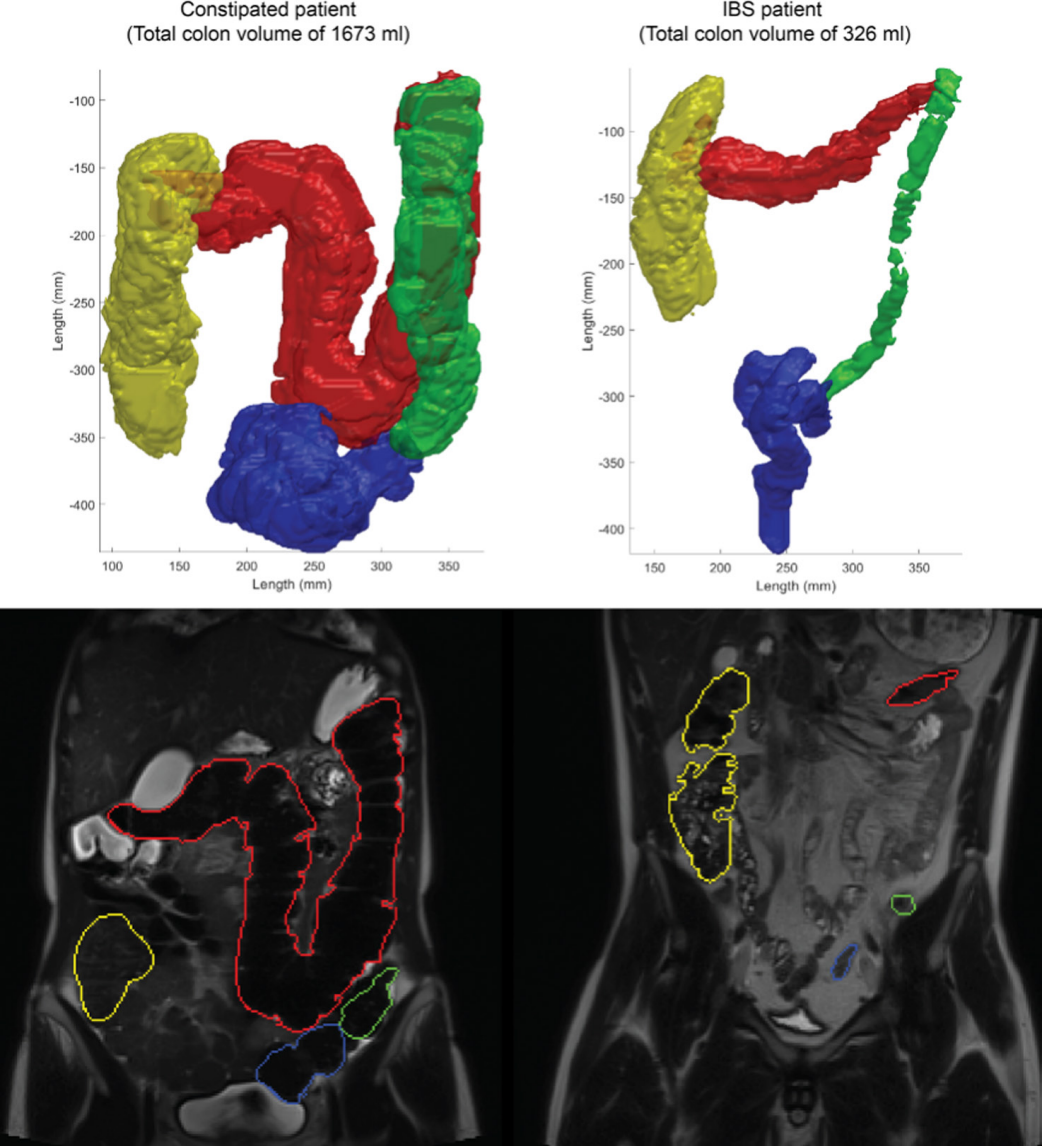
Representative MRI scans (bottom) and volume reconstructions (top) demonstrating the segmental volumes from a patient with functional constipation (to the left) and a patient with irritable bowel syndrome, constipation type (to the right)
